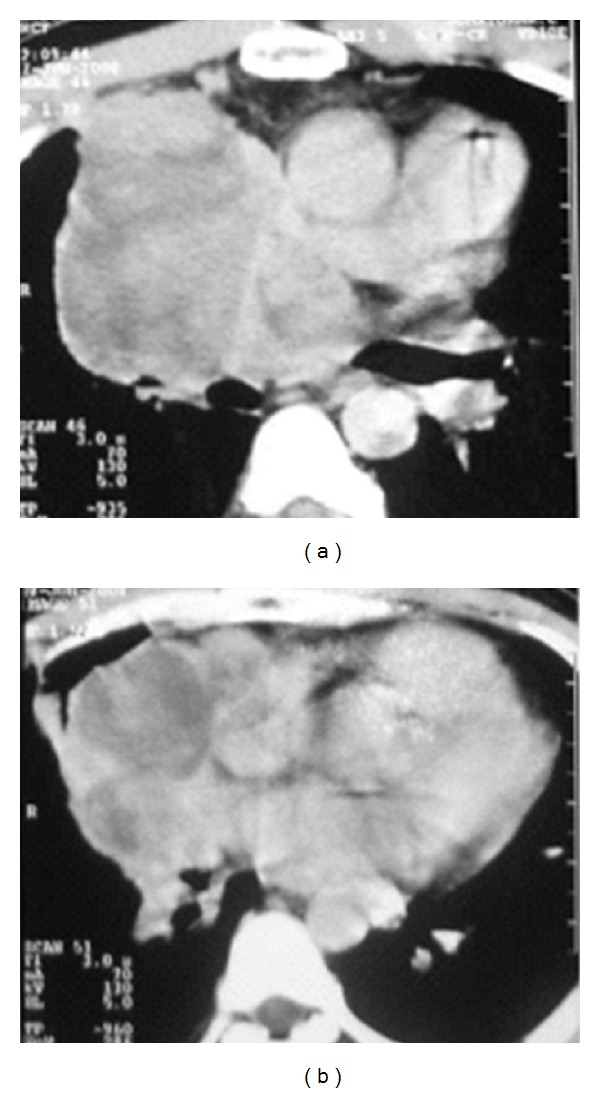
Axial post-contrast CT scan of the chest showing areas of necrosis in the mass with adherence to the pericardium.
